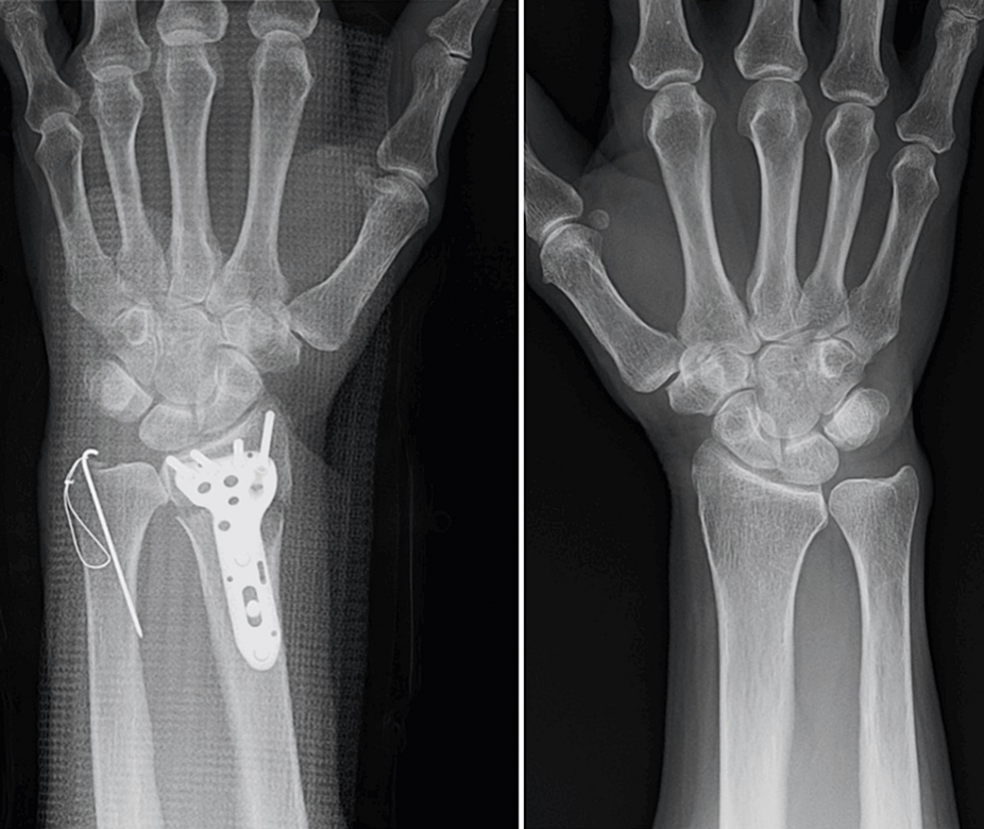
Posteroanterior radiographs of the affected side after the surgery and the intact side.
